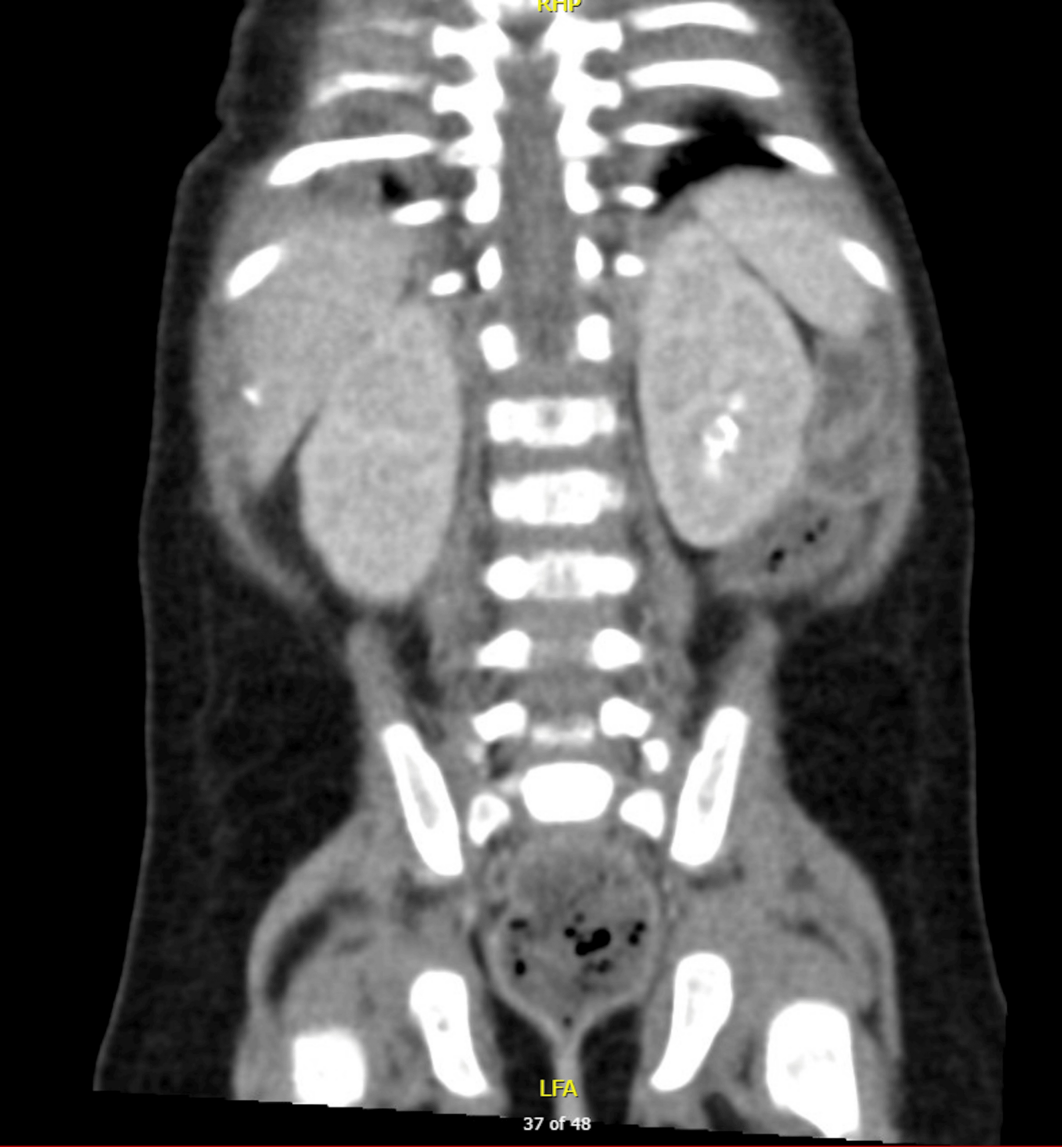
CT of the abdomen (bladder view). Coronal views of the patient’s abdominal CT showing a distended bladder and stomach.
